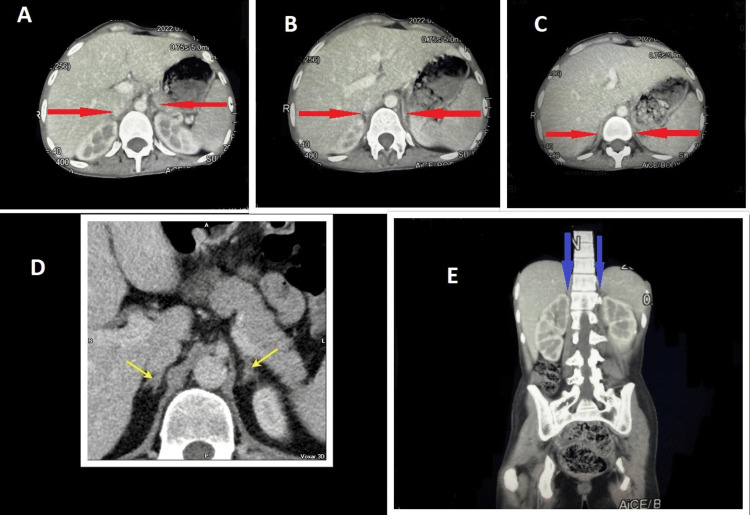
CT scan of the abdomen with contrast A-C: Multiple axial sections showing atrophied adrenal glands, not being visualized clearly (arrows). D: Normal CT anatomy of adrenal glands. The image is taken from Radiopaedia for comparison [6]. E: Glands not visible at their normal anatomic position, indicating atrophy.
